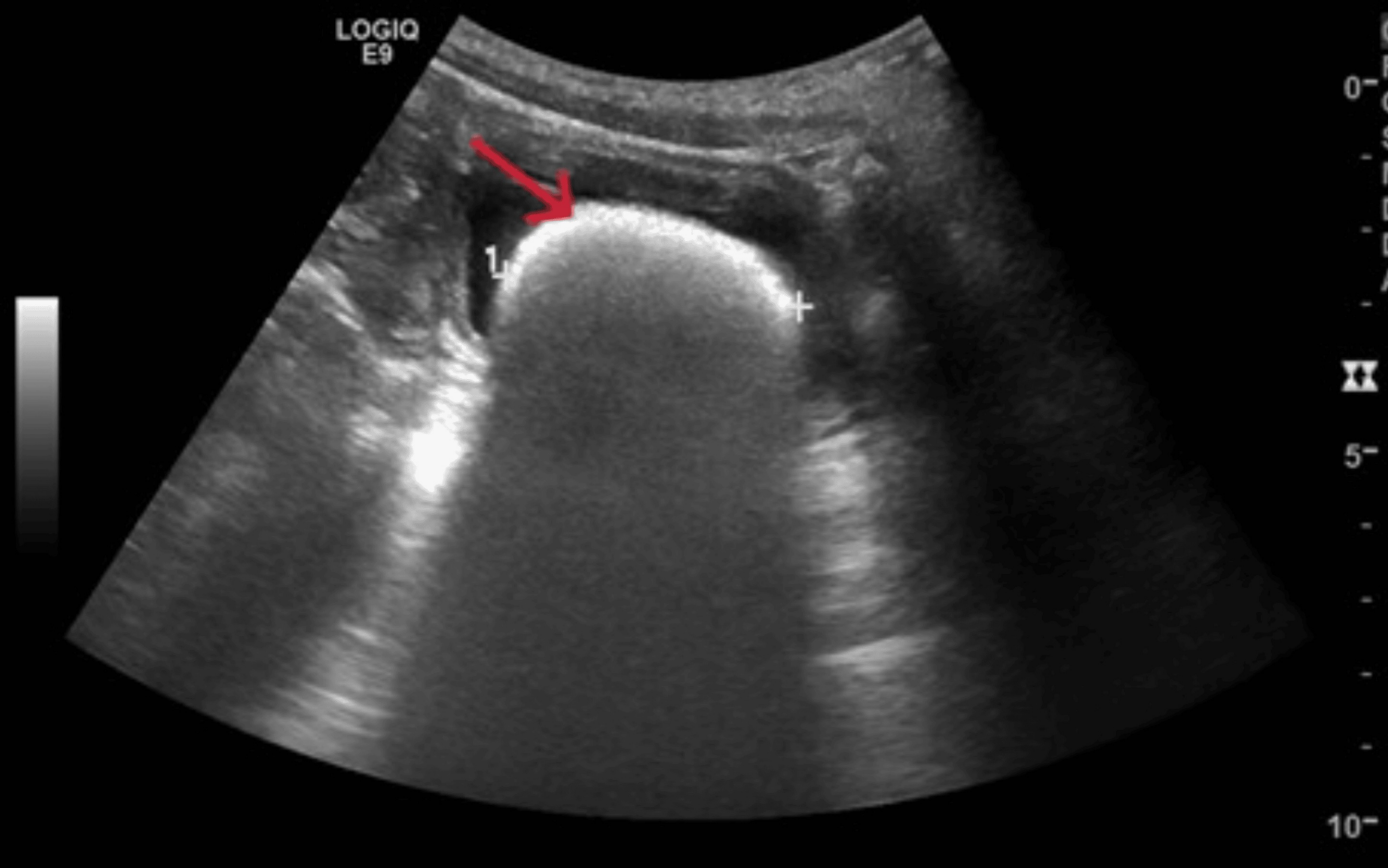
A renal ultrasound reveals a radiopaque stone in the patient’s bladder. The red arrow highlights the stone and the cross highlights the diameter of the stone.
